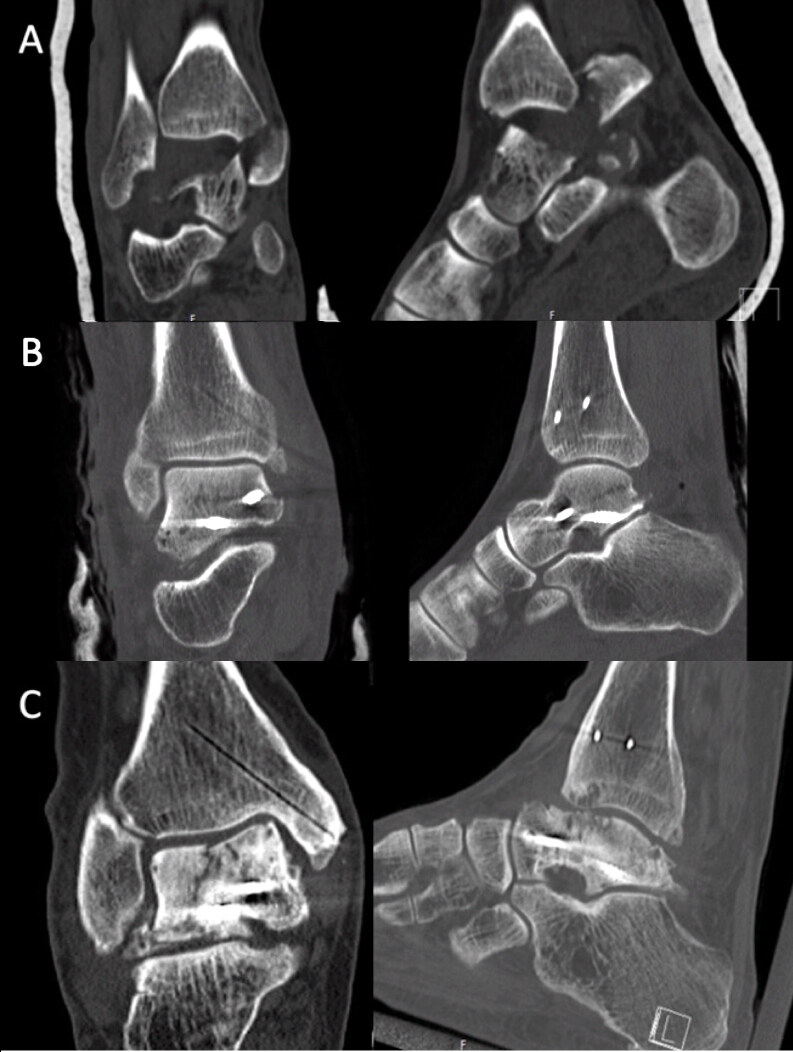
CT demonstrating a Hawkins type 3 fracture of the talus and associated medial malleolus fracture (A) in a 61-year-old man after a crush injury suffered during forest work. The fracture was treated with screw fixation (B), non-weight-bearing cast immobilization, and alendronate for 6 months. At 5.8 years after the surgery, the fracture had healed and the subchondral bone in the talar dome showed fragmentation due to collapse (C). Despite having poor ankle function (SEFAS of 12), the patient declined further surgery.
